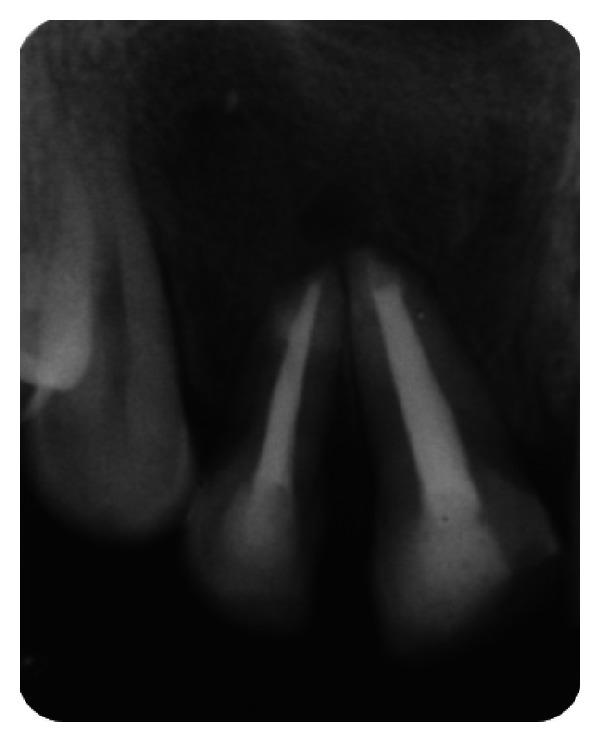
12-month follow-up IOPA radiograph.
